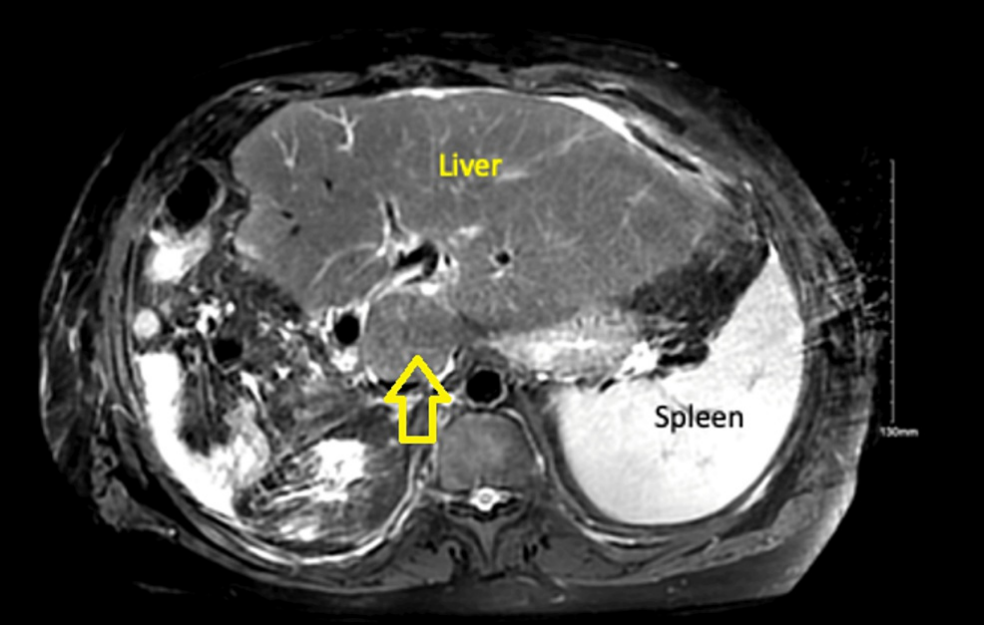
MRI abdomen with and without contrast showing compensatory hypertrophy of the left lobe of the liver with post-operative changes as a result of right hepatectomy and partial left lateral resection There are multiple arterially enhancing lesions representing hepatic adenomas (yellow arrow).
